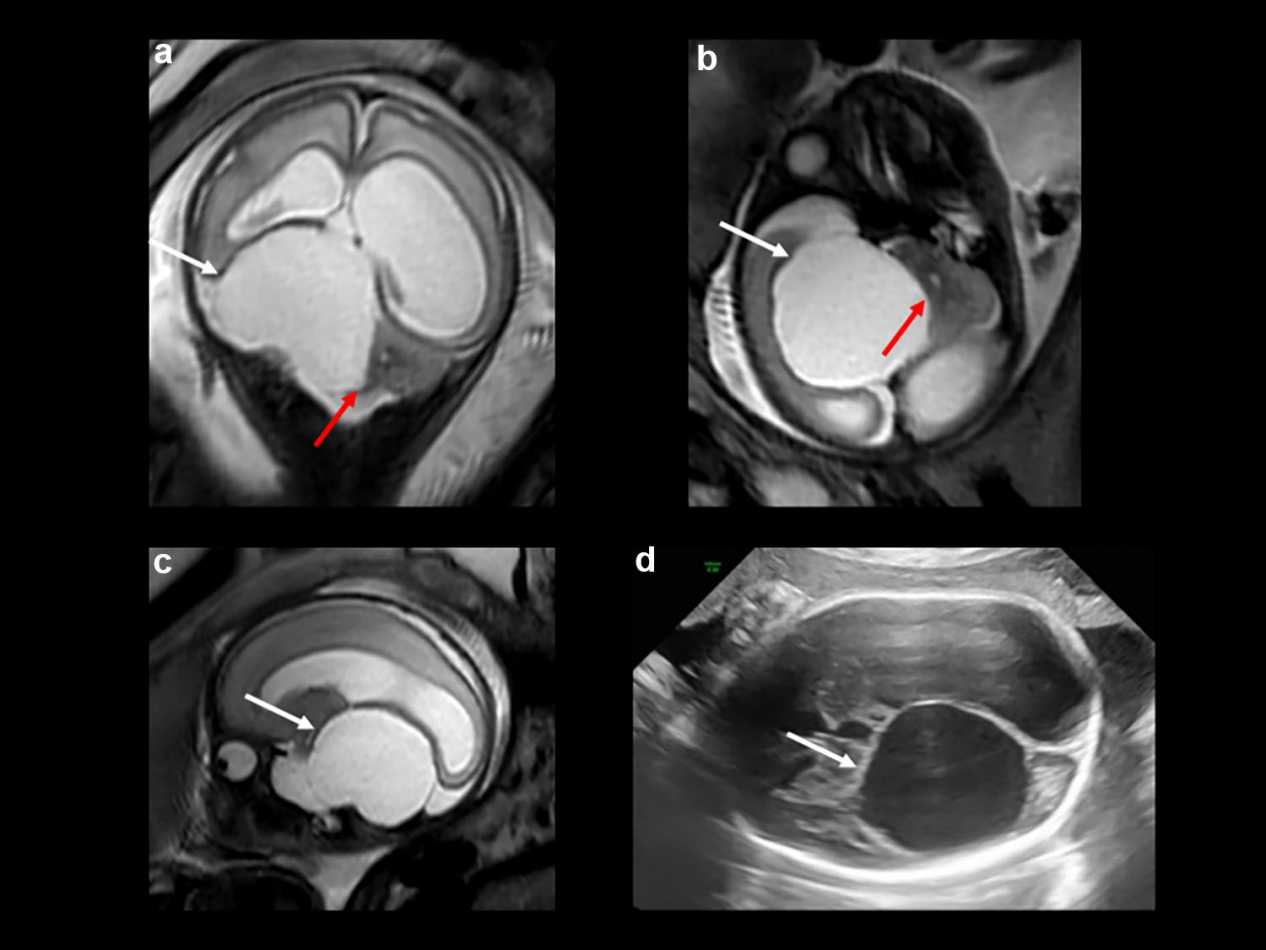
a, b, c Prenatal MRI at 27 weeks of gestational age (T2-weighted) in coronal (a), axial (b), and sagittal (c) orientations (courtesy of PD Dr. D. M. Hedderich, PD Dr. T. Finck, and Prof. Dr. J. S. Krischke, Technical University, Munich): large right-sided cystic formation with supratentorial and infratentorial extension (white arrows) with significant displacement of the right cerebellar hemisphere (a, b red arrows) and supratentorial hydrocephalus. d Prenatal ultrasound at 30 + 3 weeks of gestational age showing a large arachnoid cyst (white arrow) in the right posterior fossa with supratentorial extension and displacement of the cerebellum, mesencephalon, and compression of the aqueduct with consecutive development of a pronounced hydrocephalus
